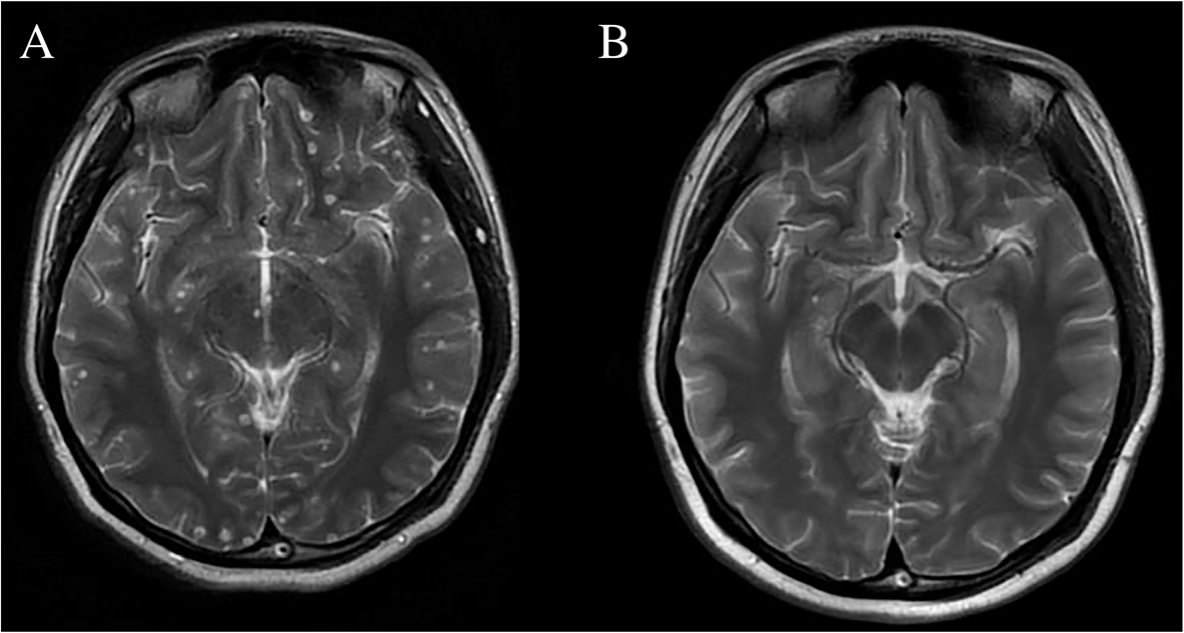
Comparison of neurocysticercosis lesions in the human brain before and after anti-parasitic treatment. a T2-weighted images showed numerous viable cysts before treatment. b A degenerating cyst in the right temporal lobe with most lesions disappeared after anti-parasitic treatment
